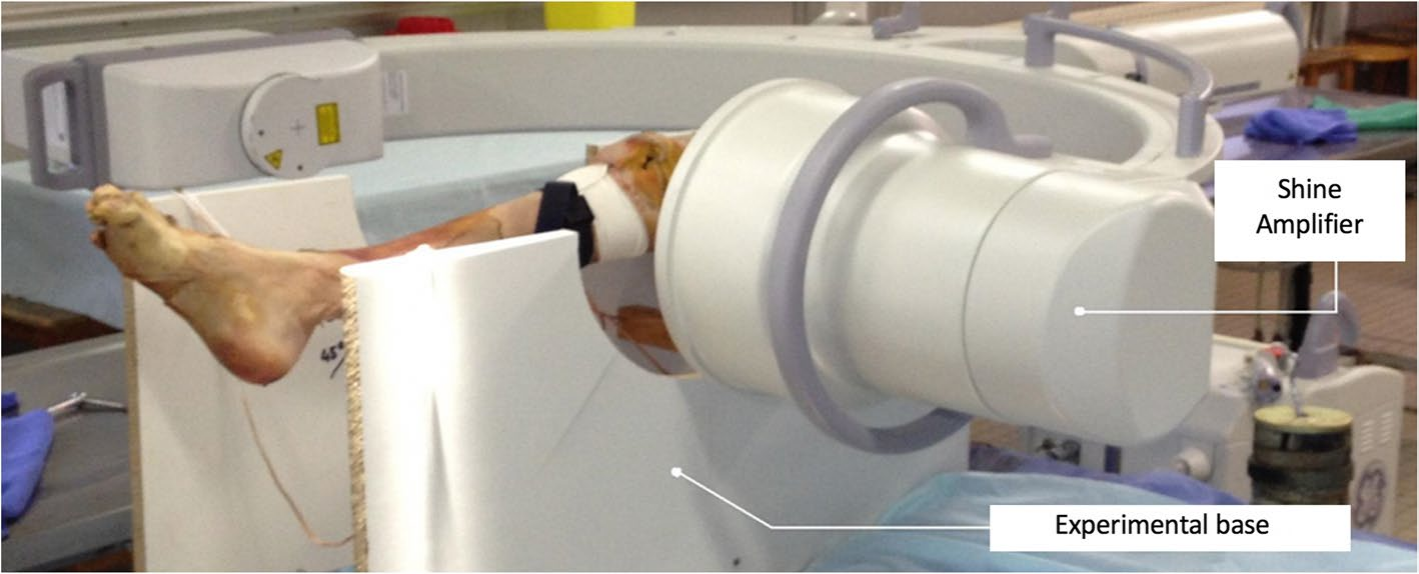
Experimental set-up and lateral radiographic image of the knee in extension with posterior stress
